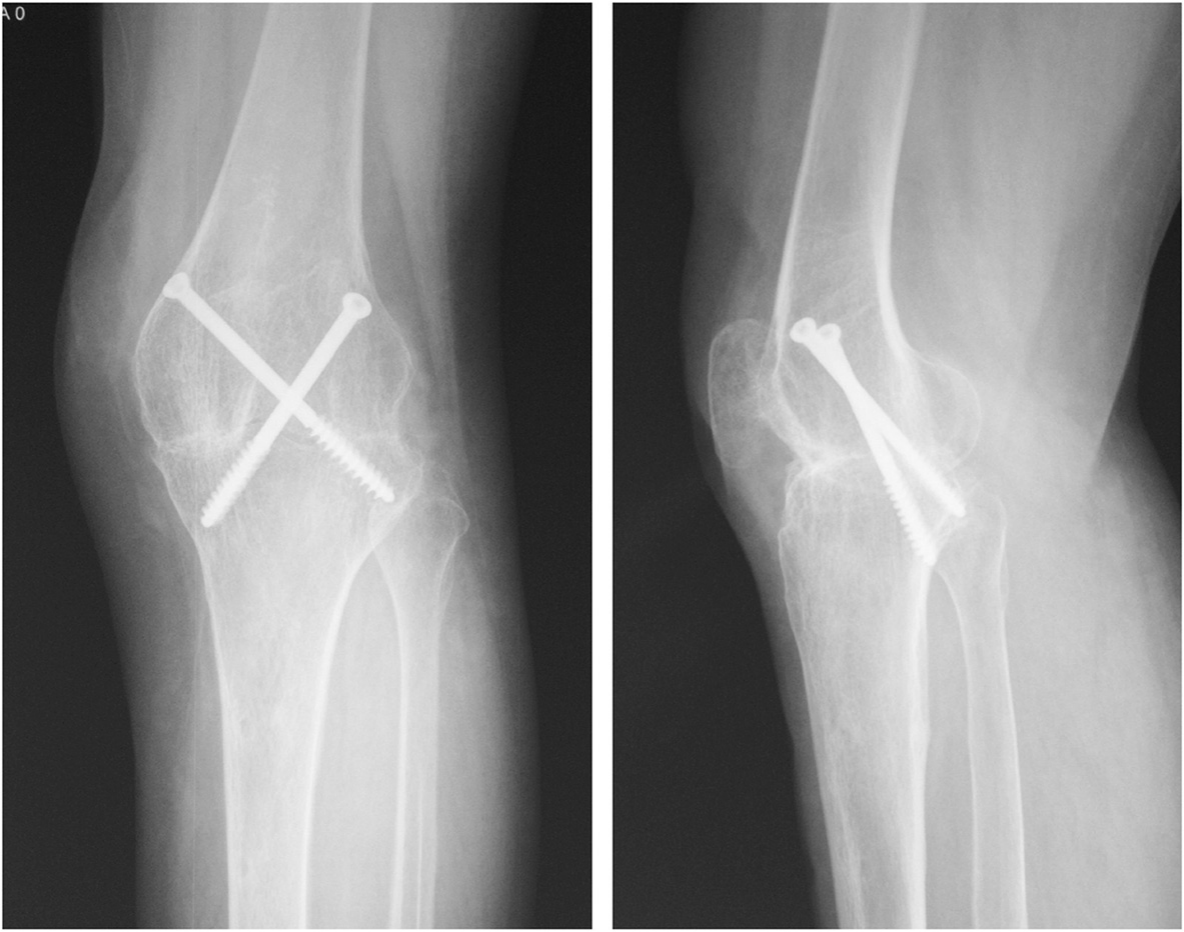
Good knee fusion shown on radiographs (anteroposterior and lateral) after removal of the external fixator
